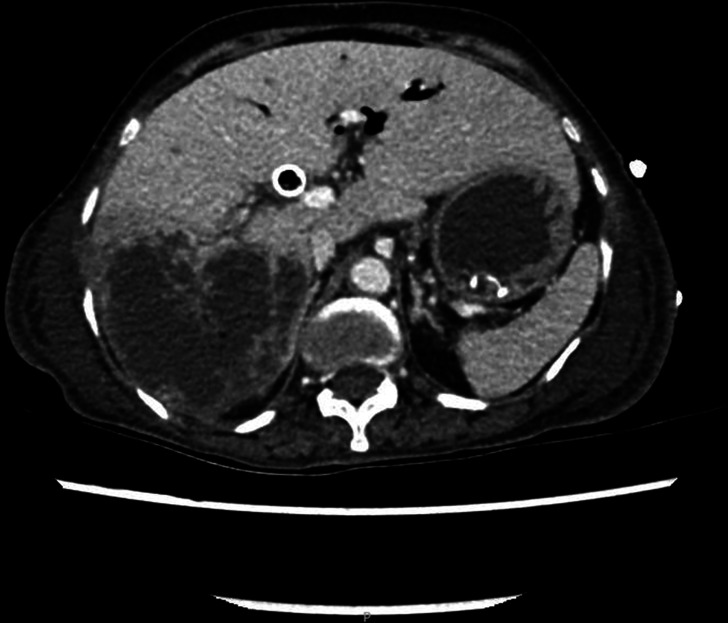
Computed tomography scan of the chest, abdomen and pelvis revealing a large (8.6×8.5×14 cm) multi-septate liver abscess within the right lobe.
